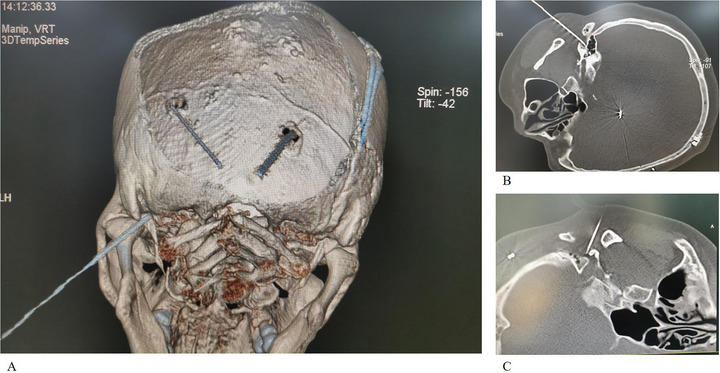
Intraoperative imaging of the first bilateral partial facial nerve radiofrequency ablation (RFA) in Case 1. (A) Three‐dimensional (3D) CT reconstruction showing the pre‐existing DBS electrodes and the radiofrequency needle targeting the left stylomastoid foramen. (B) CT‐guided needle placement at the left stylomastoid foramen. (C) CT‐guided needle placement at the right stylomastoid foramen.
